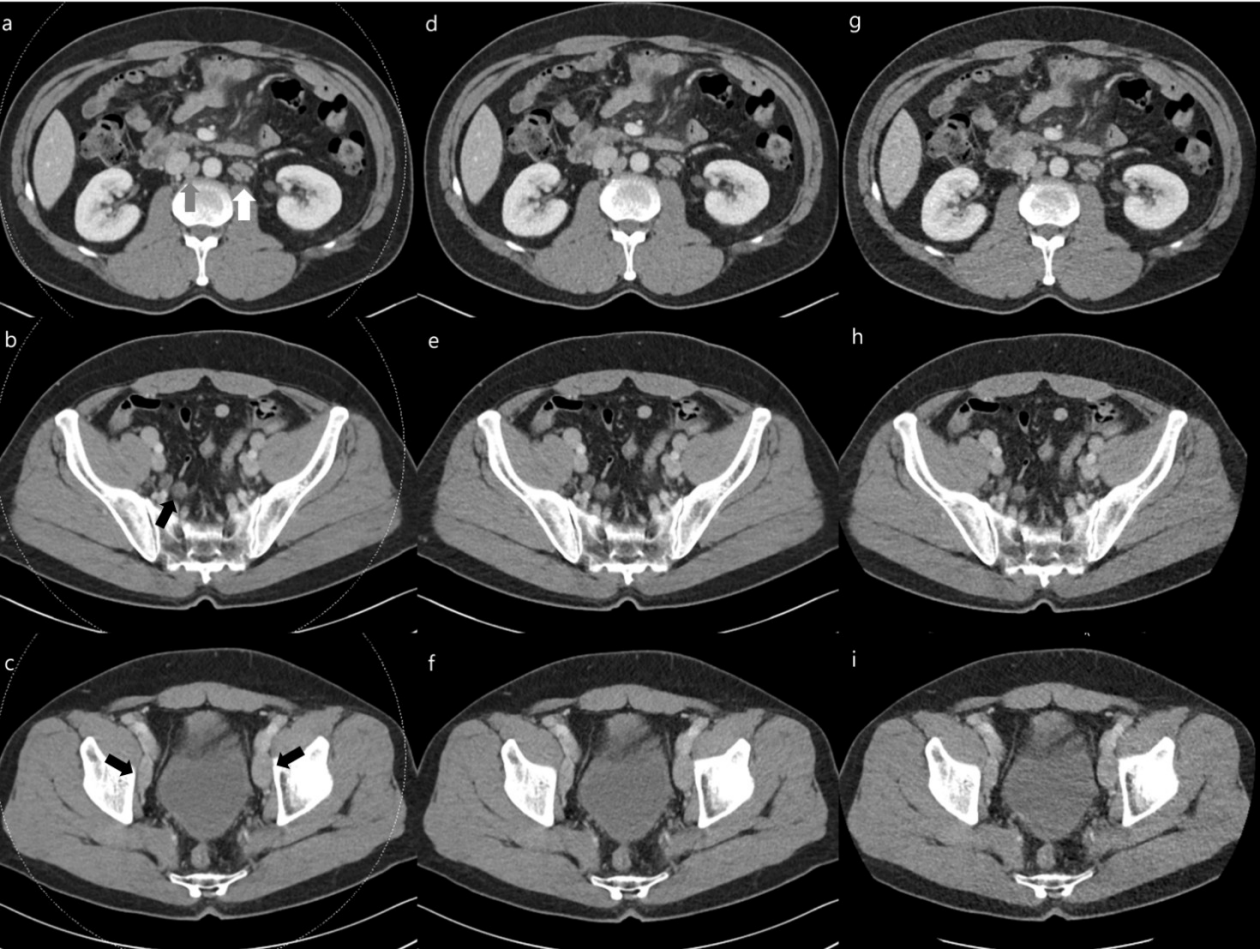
Abdominopelvic computed tomography (CT) images of a 54-year-old man with follicular lymphoma, partial response state (body mass index, 29.4 kg/m^2^; effective diameter, 27.0 cm). The three different types of CT images, acquired according to the radiation dose (a-c: standard-dose CT, 6.8 mSv; d-f: low-dose CT, 4.5 mSv; g-i: ultralow-dose CT, 2.3 mSv), show multiple enlarged paraaortic (white arrow), aortocaval (gray arrow), right common iliac, and bilateral external iliac lymph nodes (black arrows). Identical findings for the aforementioned lesions were reported by two radiologists. The overall image quality score was 4 for ultralow-dose CT images and 5 for low-dose and standard-dose CT images, according to two radiologists; the image quality score was 5 for all the three types of CT images according to one hematologist.
